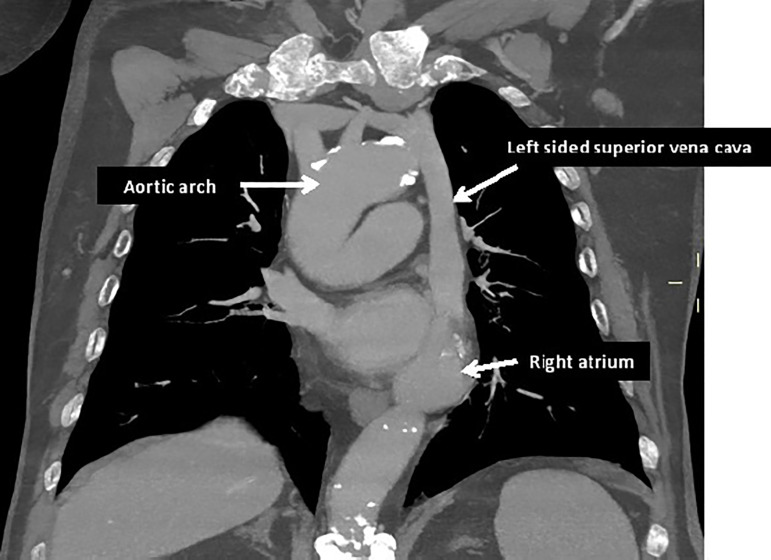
CT scan: Coronal MPR MIP image. Right IJV continues to the left where it receives the left IJV to form a left sided superior vena cava.
